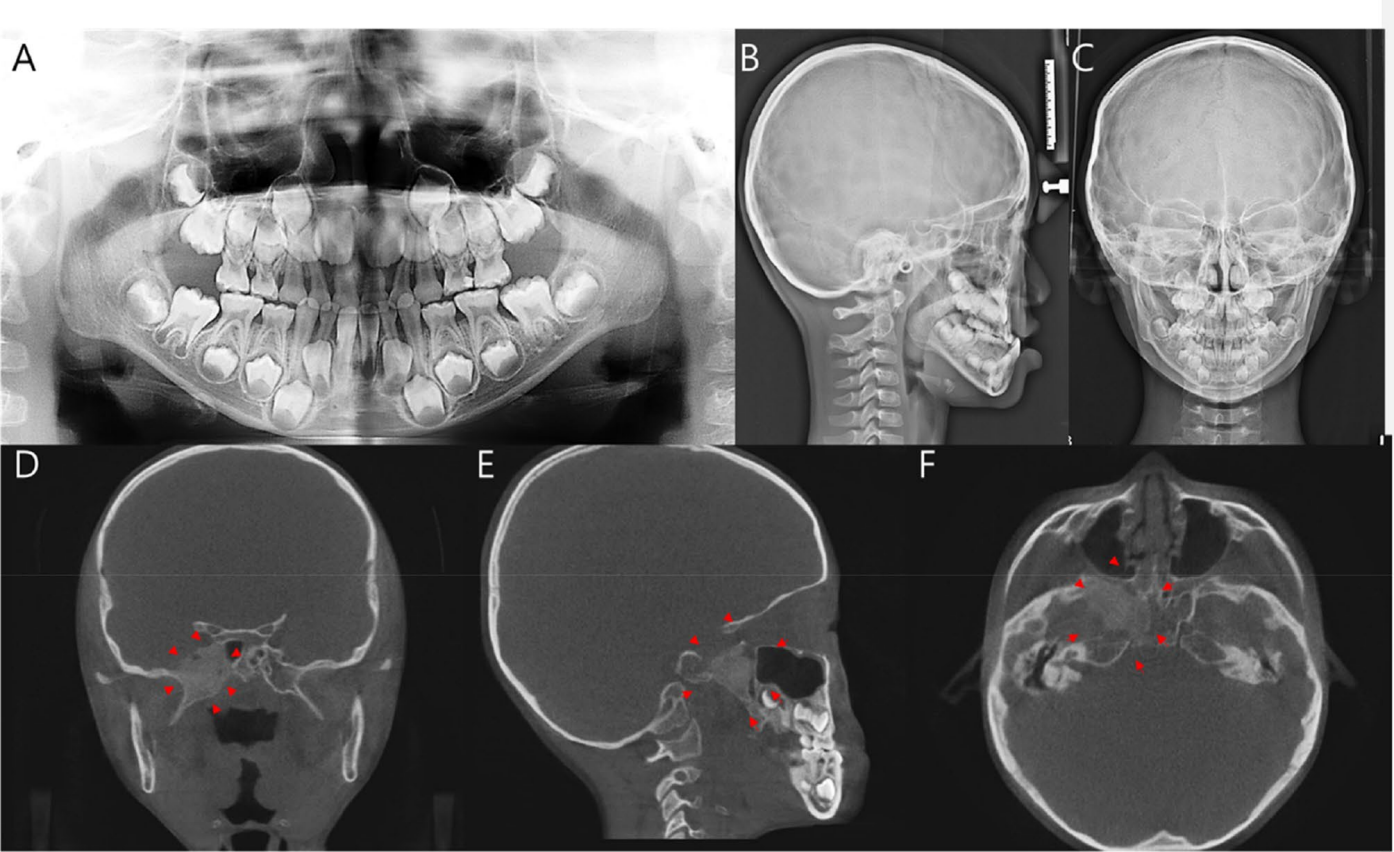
Fibrous dysplasia of the right sphenoid sinus was found on the 3D CBCT images of a 6-year-old male patient. The cortex had been thinned but was still intact, and granular internal bone pattern was observed on CBCT images, while no abnormal structures found on 2D radiographic images. (A) Panoramic radiograph, (B) lateral cephalogram, (C) postero-anterior cephalogram, (D–F) slices of CBCT showing abnormal bone pattern.
